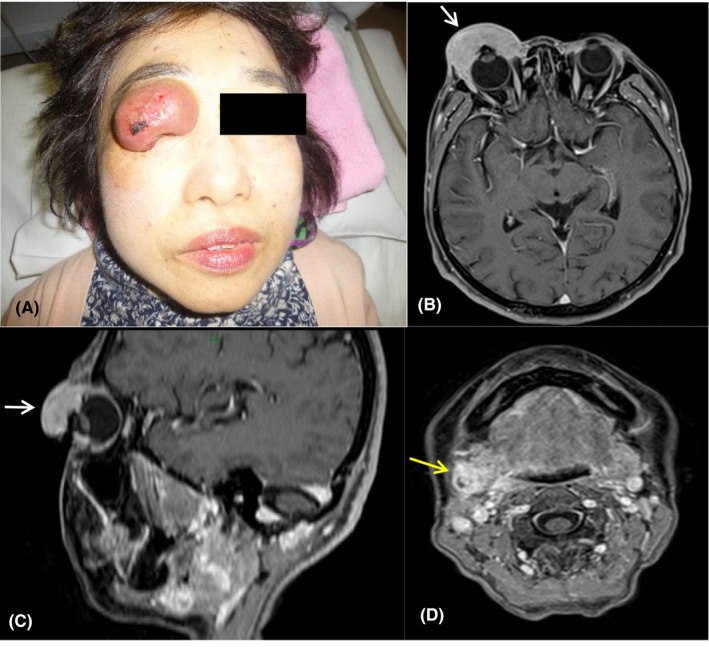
Clinical photograph of the primary tumor of the right upper eyelid (A). The right upper eyelid was replaced by a pinkish tumor with a slightly ulcerative surface showing a small amount of bleeding. Pretreatment axial MRI image (B), sagittal image (C) of the primary tumor. Right submandibular lymph node metastasis (D) (arrows)
